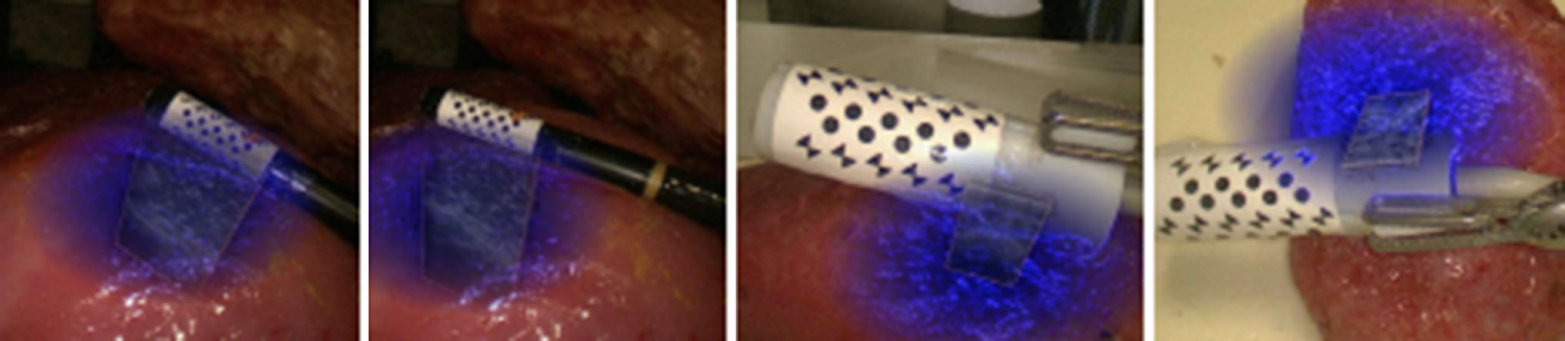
Ultrasound image is fused with laparoscopic image using an inverse realism technique [19]
